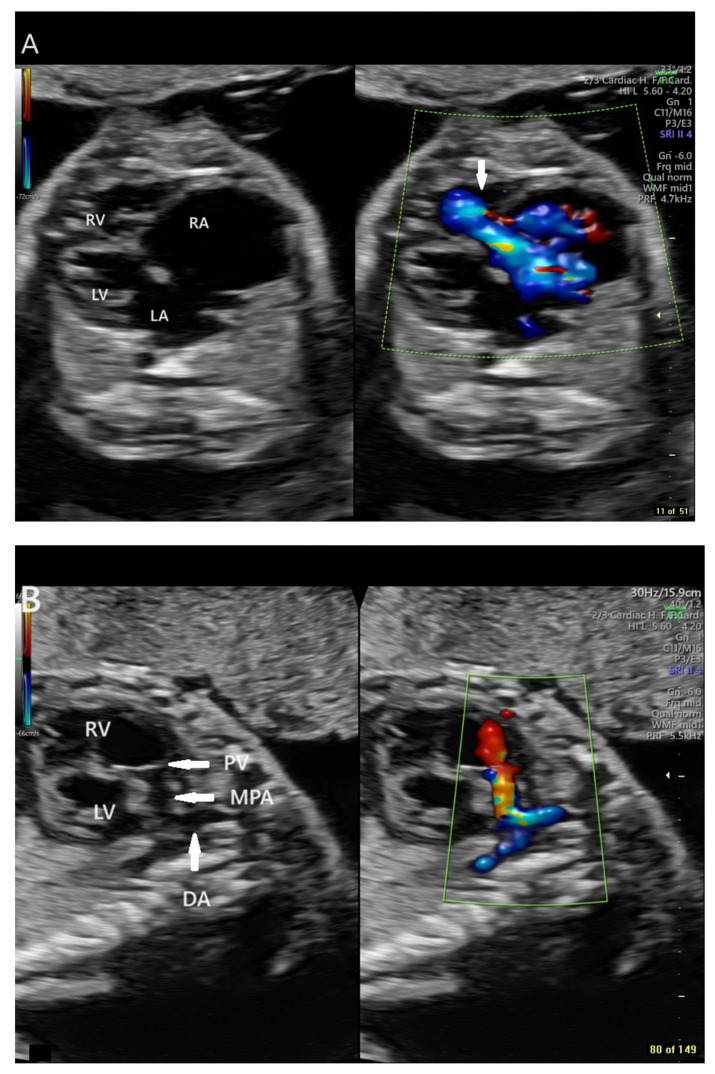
Panel (A): Paired fetal echocardiographic apical still frame images showing cardiomegaly, right atrial dilation, tricuspid annular dilation, and right ventricular enlargement at presentation at 28 6/7 weeks gestation. RA = Right Atrium, RV = Right Ventricle, LA = Left Atrium, LV = Left Ventricle. Doppler Color flow mapping superimposed on the panel at right demonstrates severe tricuspid insufficiency (arrow). Panel (B): Paired fetal echocardiographic sagittal still frame images of the dilated RV, the normal-sized RVOT, and the reverse-oriented ductus. RV = Right Ventricle, PV = Pulmonary Valve, MPA = Main Pulmonary Artery, LV = Left Ventricle, DA = Ductus Arteriosus. Doppler Color flow mapping in the panel at right demonstrates retrograde flow in the reverse-oriented ductus (blue), retrograde flow in the main pulmonary artery (orange), and marked pulmonary valve insufficiency (red). All images were produced on a GE Voluson E10 Ultrasound System.
